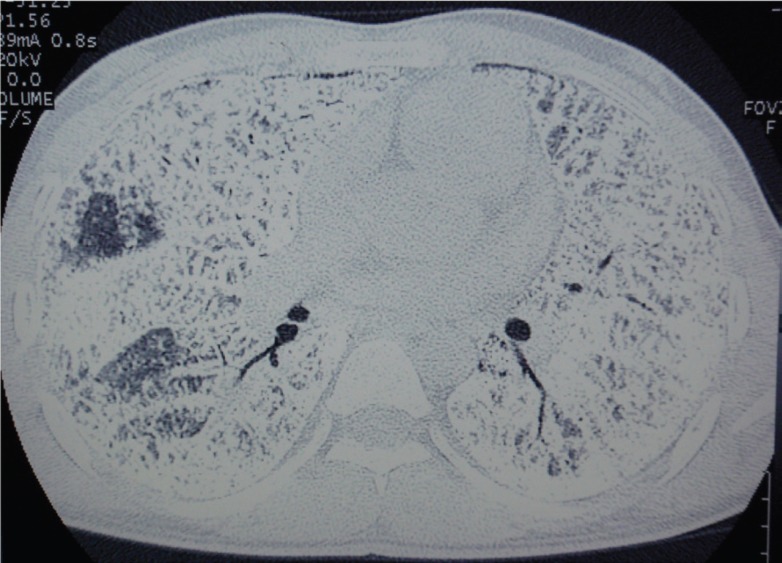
This high-resolution computed tomogram chest demonstrates diffuse intra-alveolar opacities of calcific density in bilateral lung parenchyma, septal thickening, and black pleural lines along with calcification along the interlobar septa and subpleural regions
